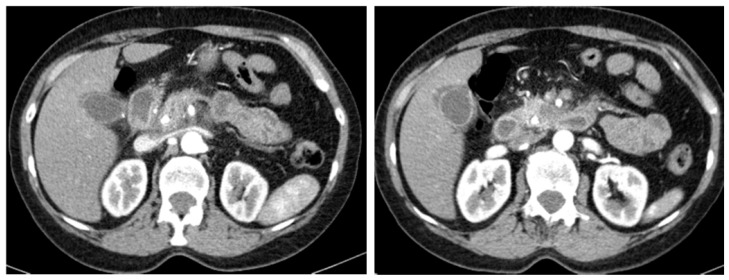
CT examination (pancreatic phase) of ductal adenocarcinoma of the pancreatic head with the presence of the perivascular cuff surrounding the superior mesenteric artery.
